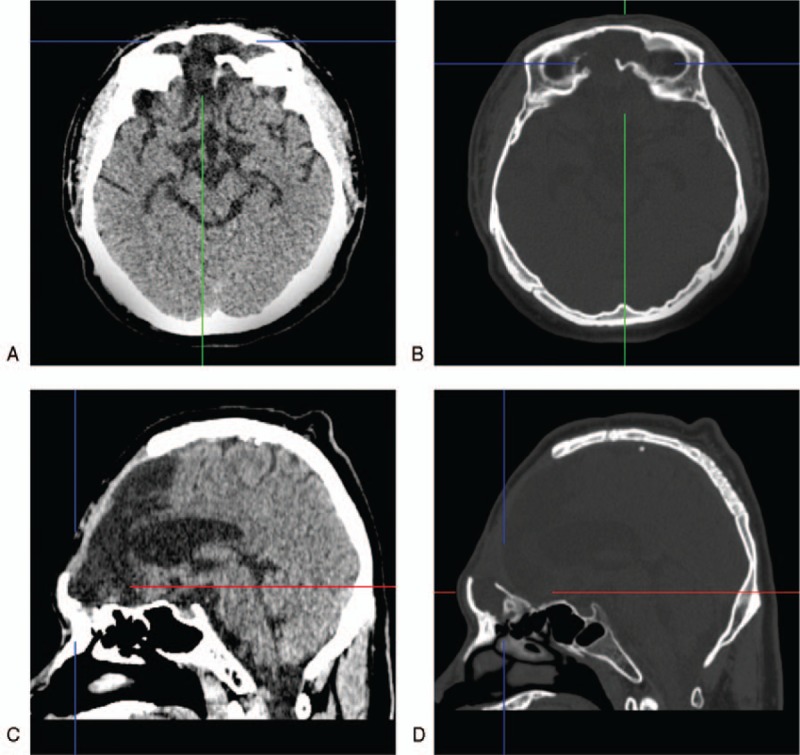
Thin-slice CT images. A–D: The axial and sagittal CT revealed the open frontal sinus. Low-density liquid-filled areas are visible in the opened frontal sinus. CT = computerized tomography.
